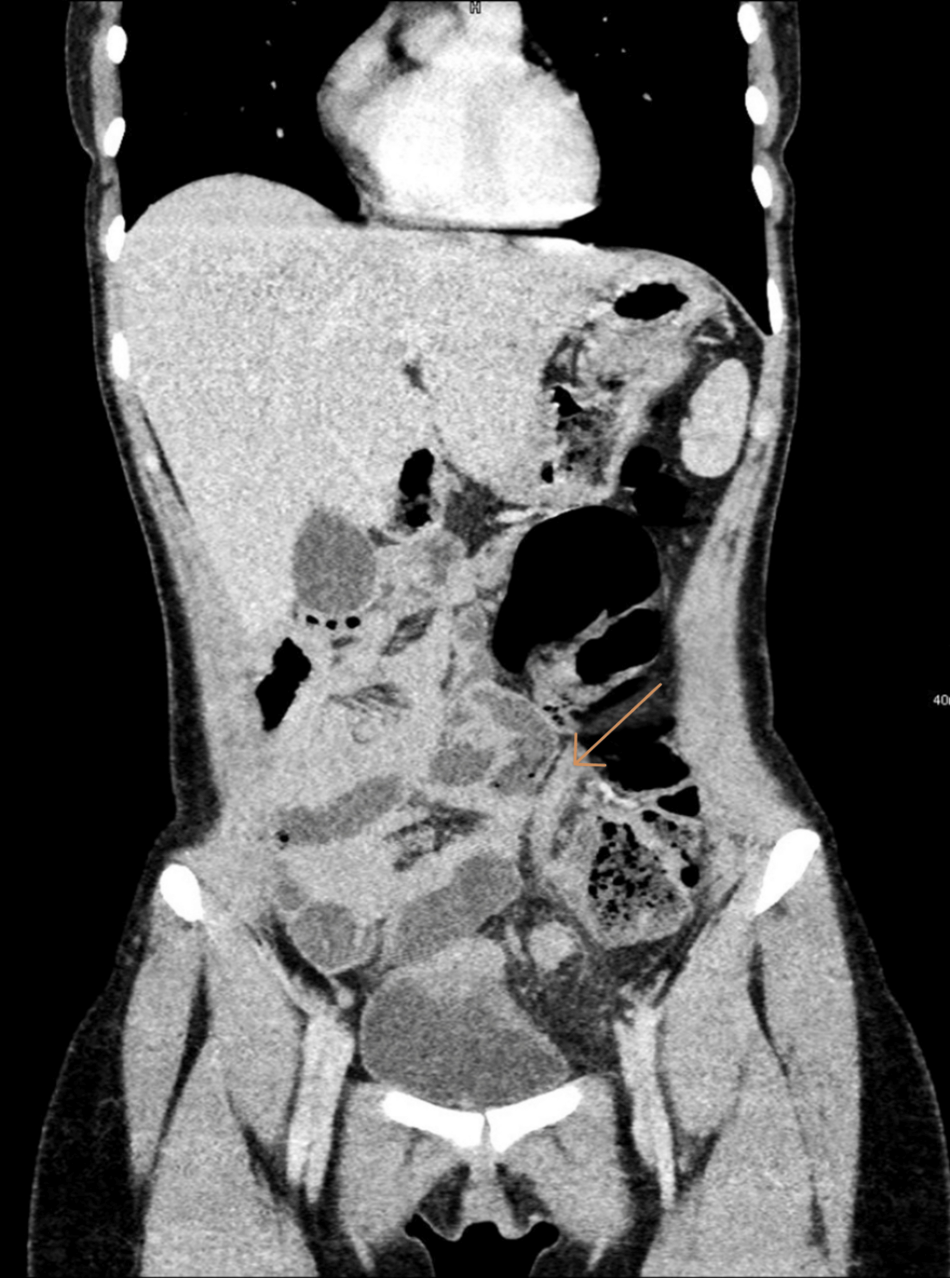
Coronal CECT demonstrating the appendix in the left lower quadrant (arrow), with the cecum and terminal ileum located abnormally on the left side. The SMV is seen left of the SMA, consistent with intestinal malrotation. Localized inflammatory changes are present without perforation or abscess formation. The arrow indicates the distal appendiceal tip. CECT: Contrast-enhanced computed tomography; SMA: superior mesenteric artery; SMV: superior mesenteric vein.
